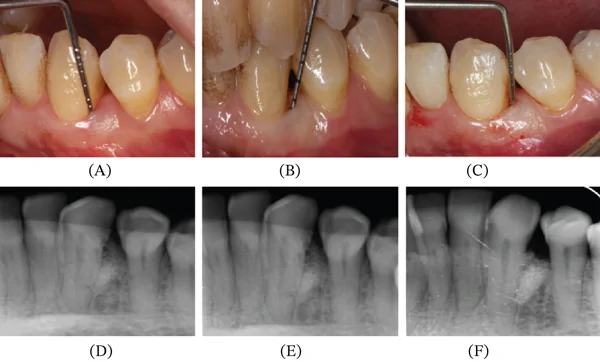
(A) At 6 months probing depth, (B) 12 months probing depth, (C) 24 months probing depth, (D) 6 months radiographic evaluation, (E) 12 months radiographic evaluation, and (F) 24 months radiographic evaluation.
